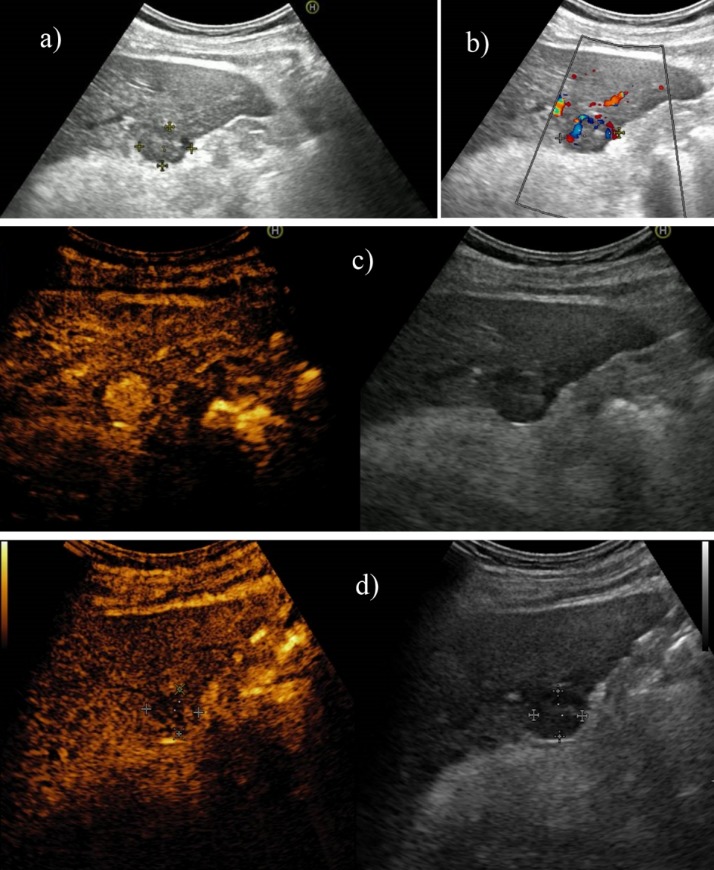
In a 56-year-old patient, standard ultrasound showed a small nodule of 1,8 cm in the hepatic segment V
